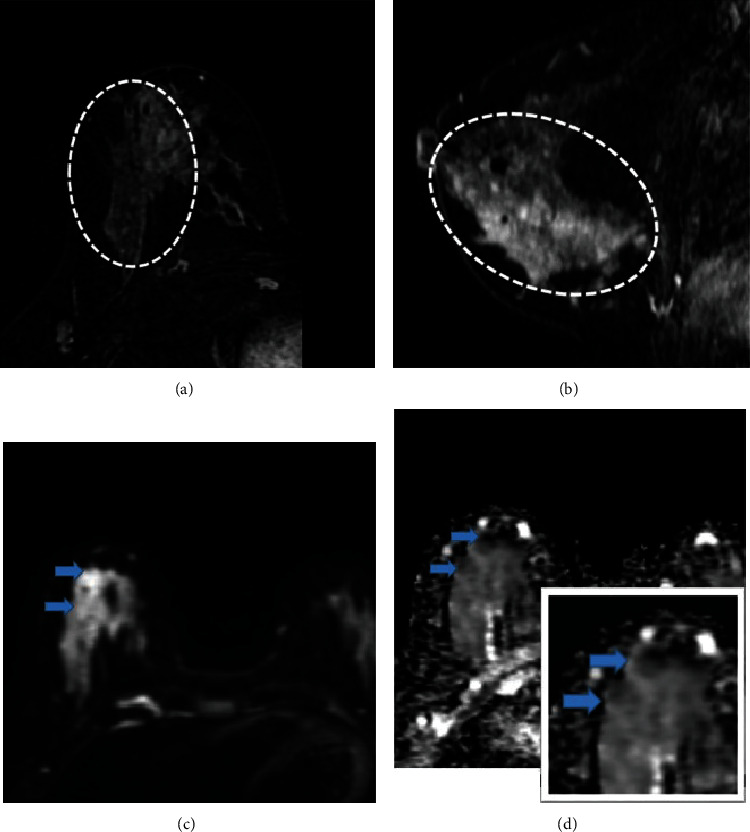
(a, b) Axial and sagittal contrast-enhanced breast MRI images in a 47-year-old female with pathology-proven invasive ductal carcinoma and ductal carcinoma in situ, depicting segmentally distributed clumped nonmass enhancement (dashed circle) in the right breast. (c, d) DWI and ADC images show heterogenous restriction which is better demonstrated in the inset image.
